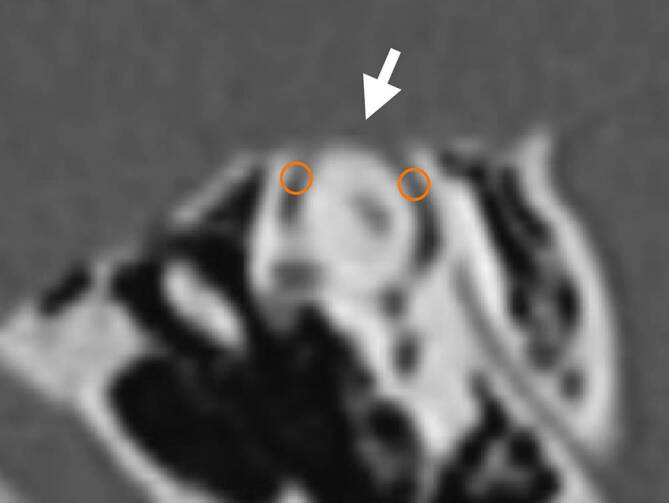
Dehiscence (*arrow*) of the superior semicircular canal on the right in an oblique coronal reconstruction (parallel to the superior semicircular canal plane, previously referred to as “Pöschl plane”) of a thin-slice spiral computed tomography data set of the temporal bone (patient 2). The two circles mark the points for opening the semicircular canal and isolating the dehiscence
